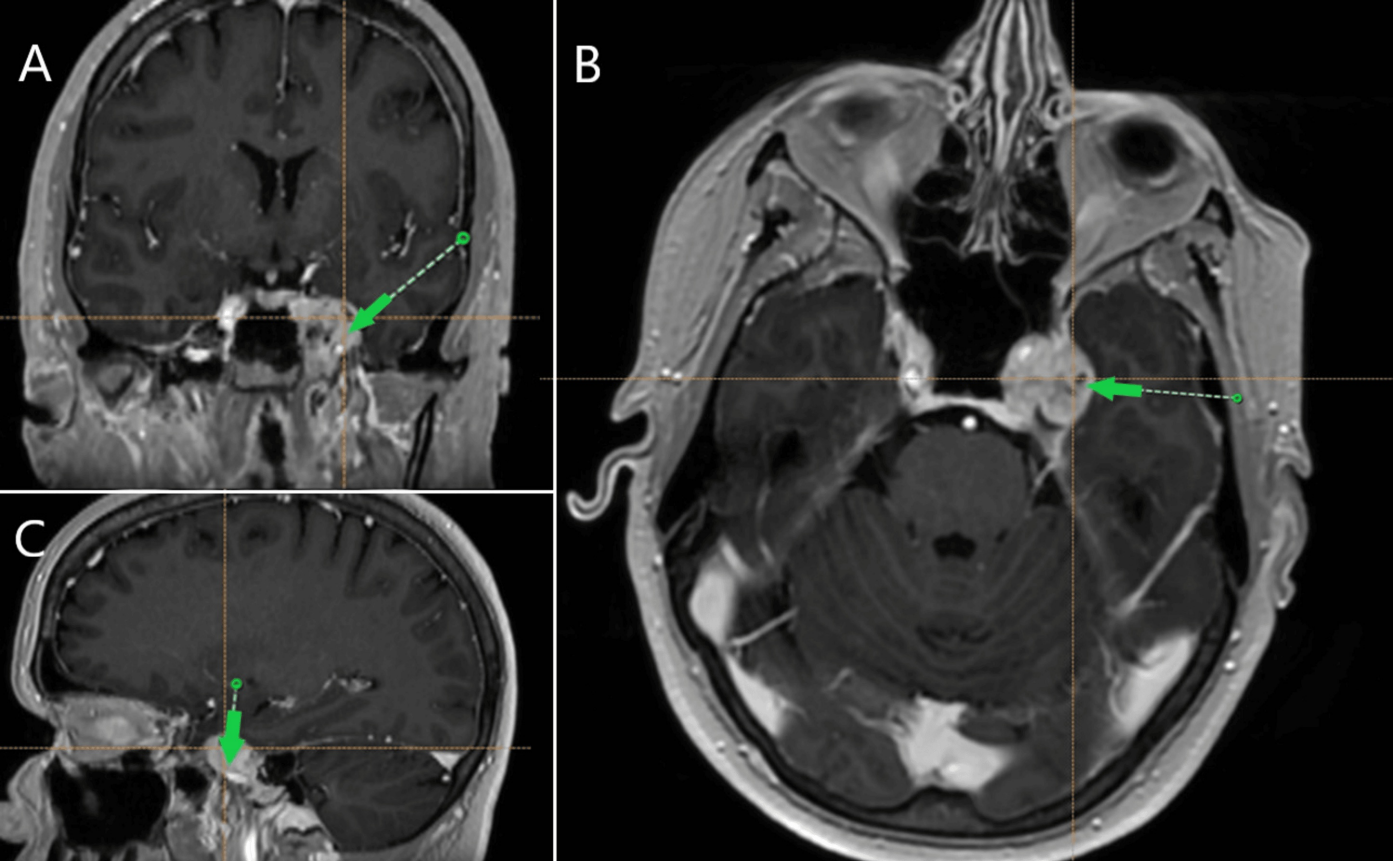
Trajectory planning Brain MRI via BrainLab® (Brainlab, Munich, Germany) with the target indicated by the green arrow at the lateral wall of the cavernous sinus on (A) coronal, (B) axial, and (C) sagittal views.
